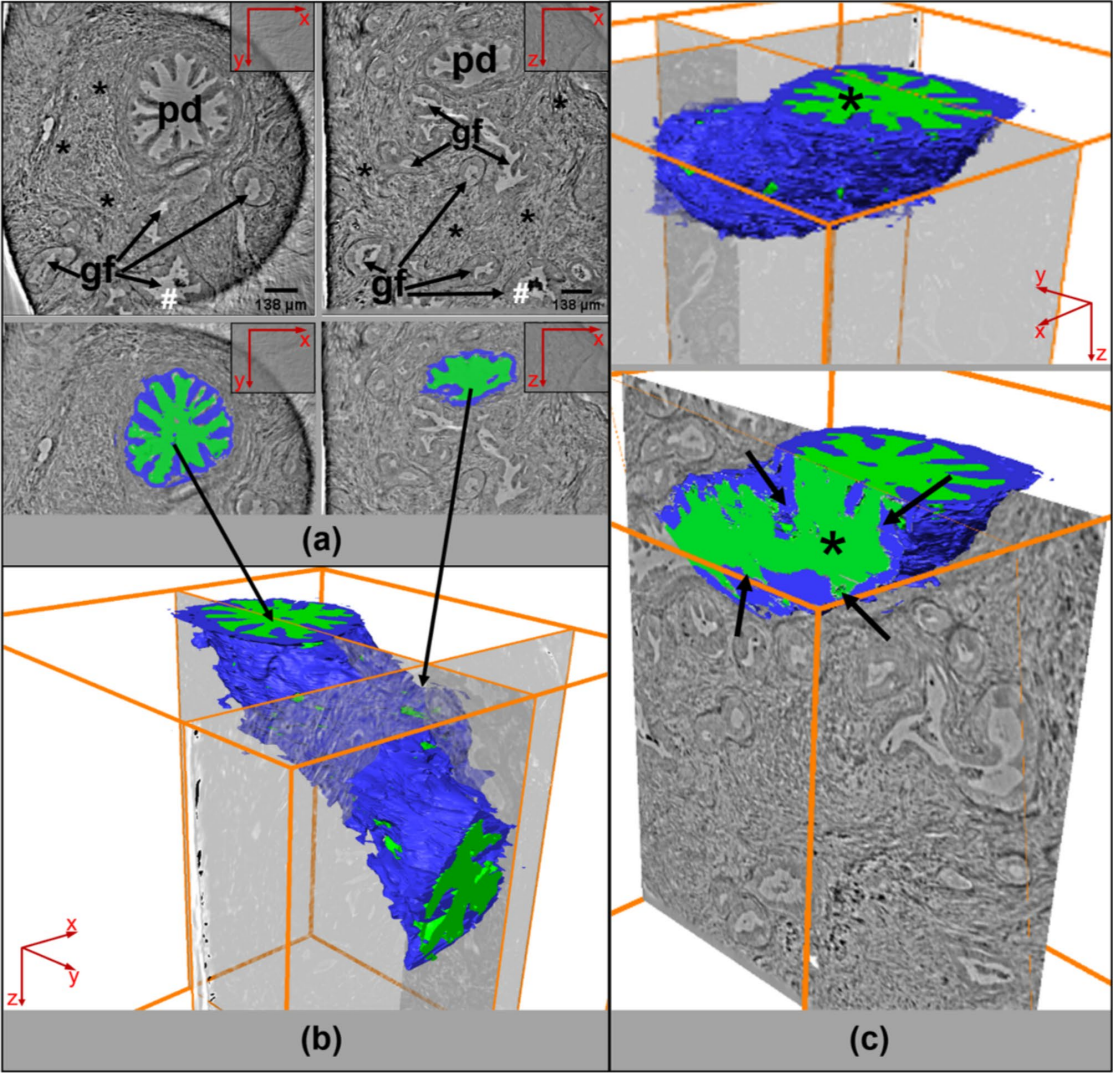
3D PCCT data set analysis of an altered pancreatic duct. Virtual sections from the X-ray tomogram of the biopsy punch from the virtual section number 42 (a, left) to a more distant virtual section number 760 (a, right) confirm the existence of altered gland formations (gf) in between the desmoplastic stroma (asterisks) as well as a star-like proliferating duct (pd) characterized by an altered epithelial morphology. Remarkably, very dense inclusions are observed within the lumina (hashtag). Red arrows in (a) indicate the displayed spatial direction of the virtual 2D tissue section. The star-like proliferating duct (pd) was semi-automatically segmented (b) through the whole volume, discriminating the lumen (green) and the epithelium (blue). The 3D reconstruction reveals the location of this duct within the biopsy volume from section 42 to the last traceable plane (b). The translucent layer marks the layer 760 in xz direction (b, right arrow). The angle of the segmented duct was virtually changed in order to evaluate a cross-section of the morphologically altered duct (c). Note, that the highly variable diameter of the lumen (c, star, green), caused mainly by the surrounding epithelium of varying height (arrows), indicates different epithelial proliferation rates in certain duct locations
